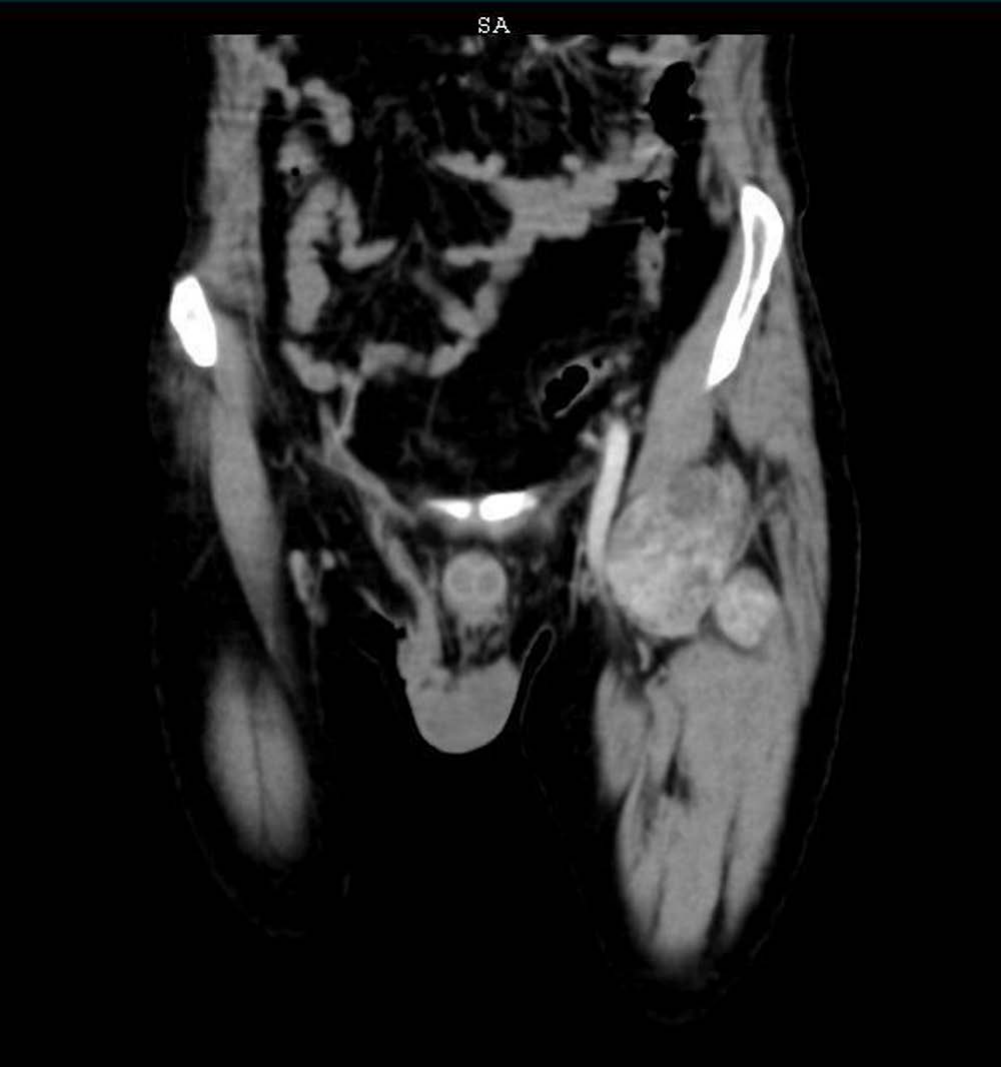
CT scan showing a well-defined mass in the top of the left thigh with well-defined margins. CT = computed tomography.
